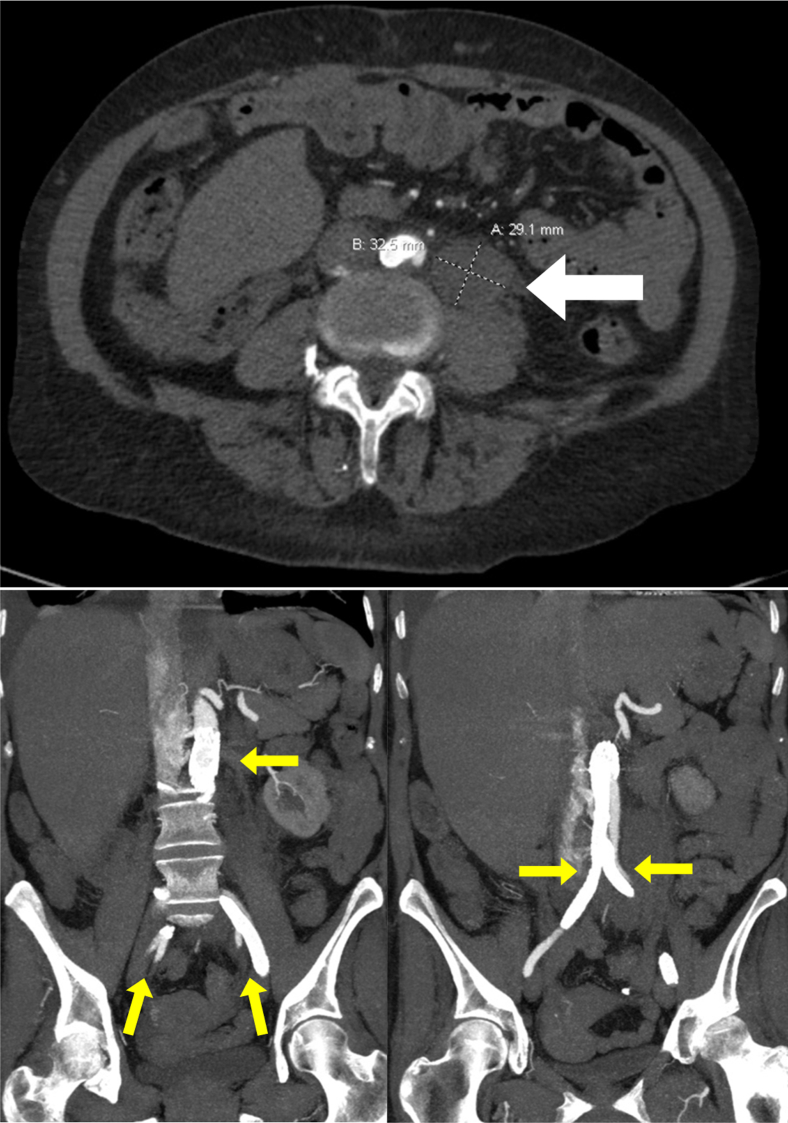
Computed tomography (CT) scan taken 1 month postoperatively demonstrating a reduction in size of a retroperitoneal hematoma (*white arrow*), as well as good perfusion through the infrarenal aorta and the bilateral common, internal, and external iliac arteries (*yellow arrows*).
